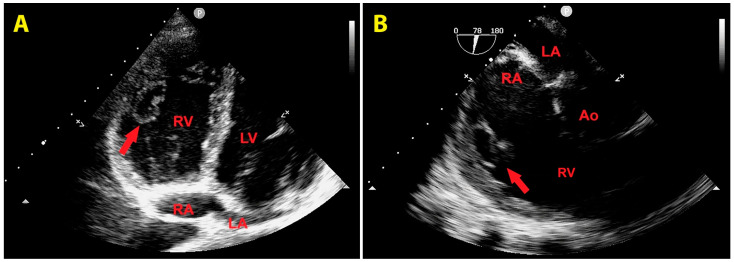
(A) Transthoracic echocardiography. Right-ventricular-focused apical four-chamber view demonstrating a slight reduction in right ventricular mass size (red arrow), characterized by an anechoic central space and a hyperechoic border. (B) Right-ventricular-focused mid-oesophageal section showing the same echocardiographic features of the right ventricular mass (red arrow), observed from the transthoracic approach. Ao, aorta; LA, left atrium; LV, left ventricle; RA, right atrium; RV, right ventricle.
